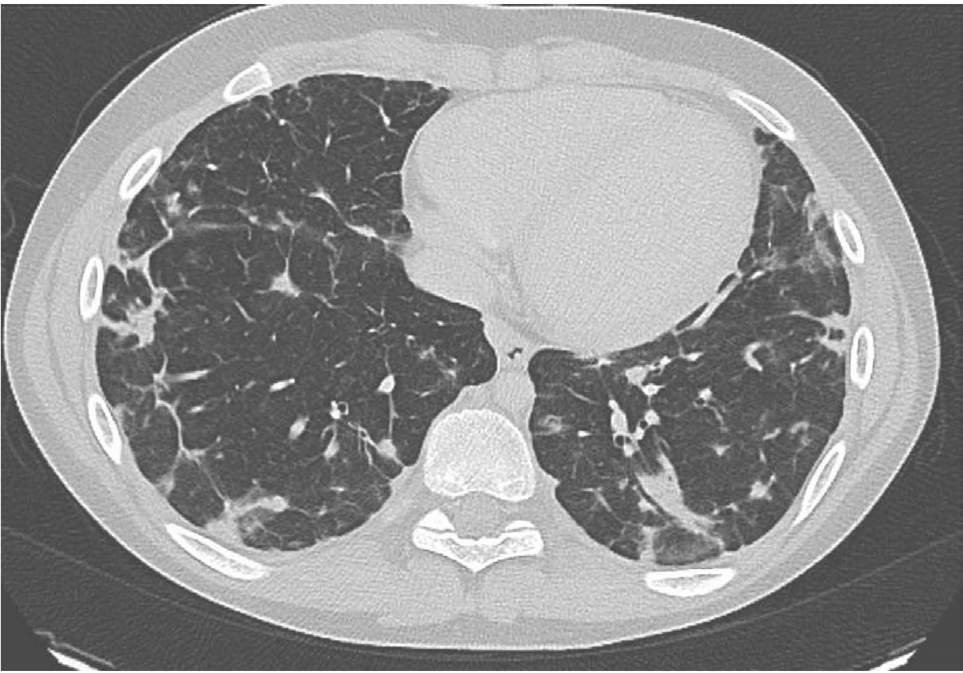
Chest CT of patient prior to treatment with mycophenolic acid showing multiple infiltrates.
